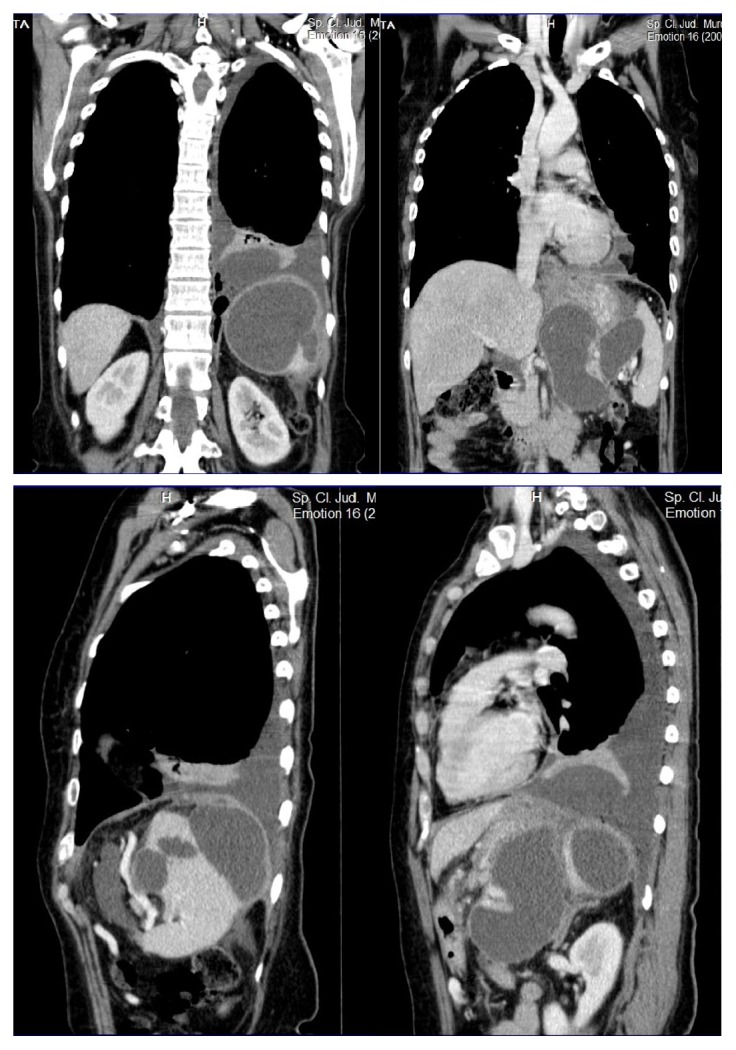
Preoperative CT scan: 3D reconstructions.
